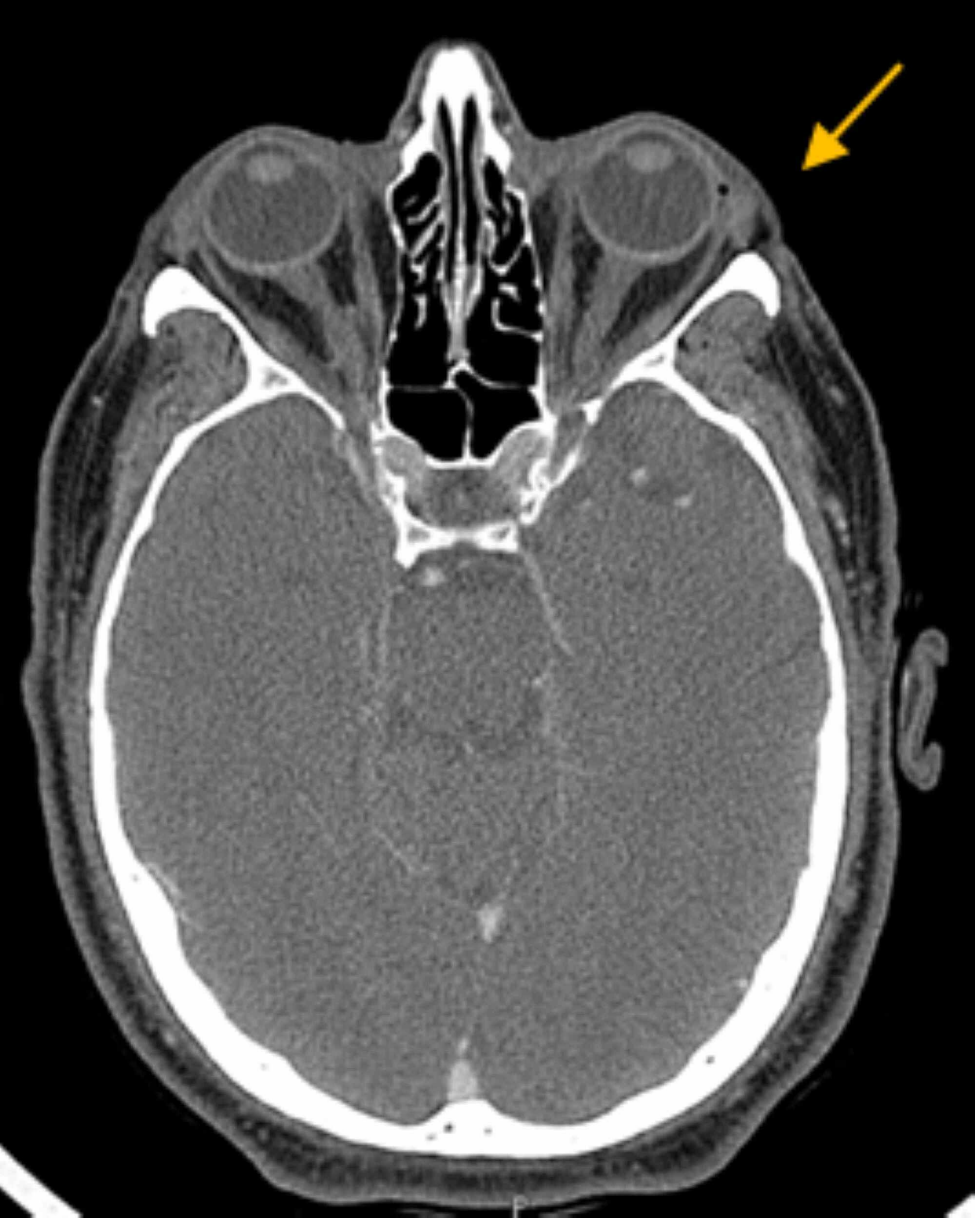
CT facial bones with contrast showing left-sided pre-septal cellulitis and probable subdermal fluid collection (yellow arrow) CT: computed tomography
